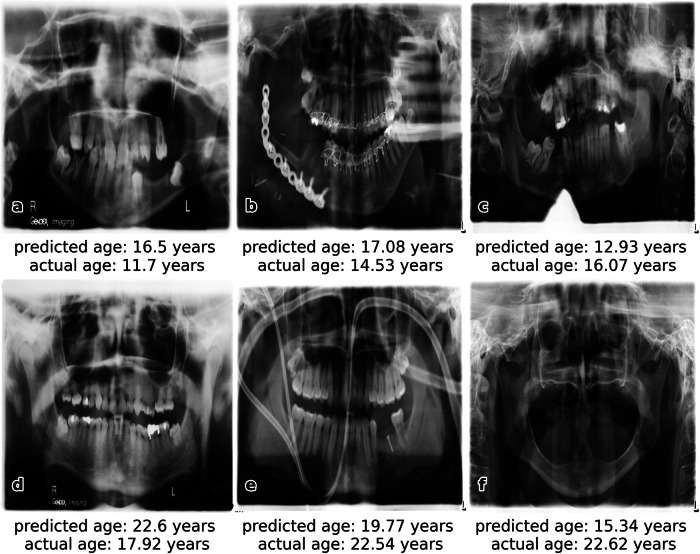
A selection of orthopantomograms (OPGs) of the test dataset with poorly predicted age. These OPGs deviate from the majority due to closed mouths with overlapping teeth (a, c), large implants (b), poor image quality, such as low contrast (a, d), the presence of medical equipment in the image (e), or the absence of teeth (f). The prediction of image (f) is the worst of all test images, presented as the red point in Fig. 2
